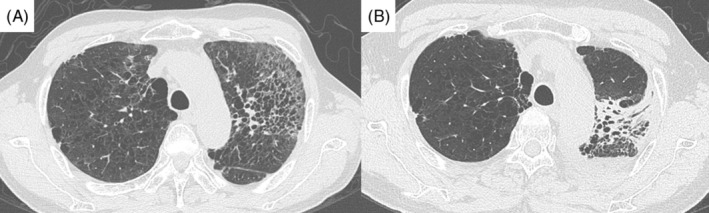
(A) Chest computed tomography scan obtained at the onset of radiation pneumonitis in April 2020. (B) A chest computed tomography scan was obtained when dabrafenib and trametinib combination therapy was initiated in August 2020.
